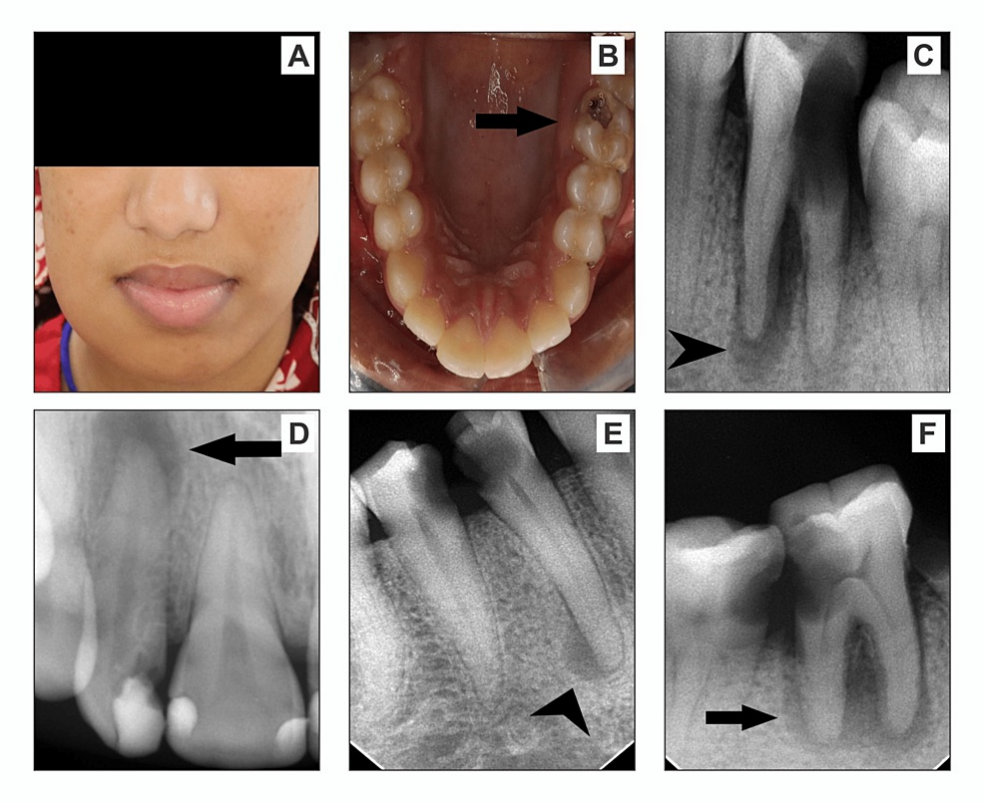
Clinical and radiographic findings of patients with periapical pathologies: (A) 19-year-old female patient presented with swelling and pain, (B) grossly carious left upper first molar with irreversible pulpitis, (C) lower first molar with carious crown and radiolucent lesion involving full length of mesial root, (D) upper lateral incisor with recurrent caries and periapical cyst, (E) lower first premolar with periapical granuloma and second premolar with the widening of the PDL space, (F) lower second molar with characteristic endo-perio lesions. PDL, periodontal ligament
